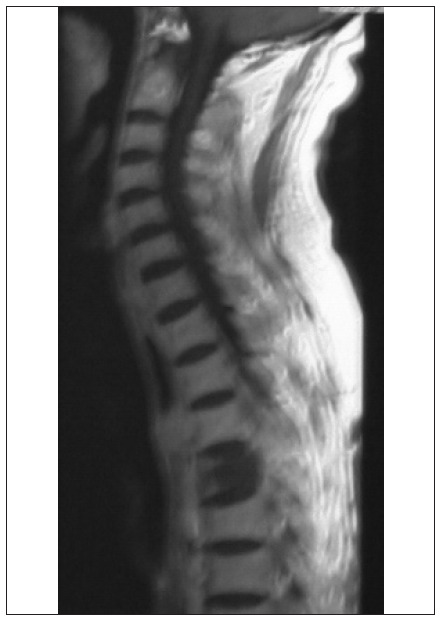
Spine T1-magnetic resonance imaging: a lytic lesion is seen in the T4 vertebral body, suggestive of metastasis from lung carcinoma.
